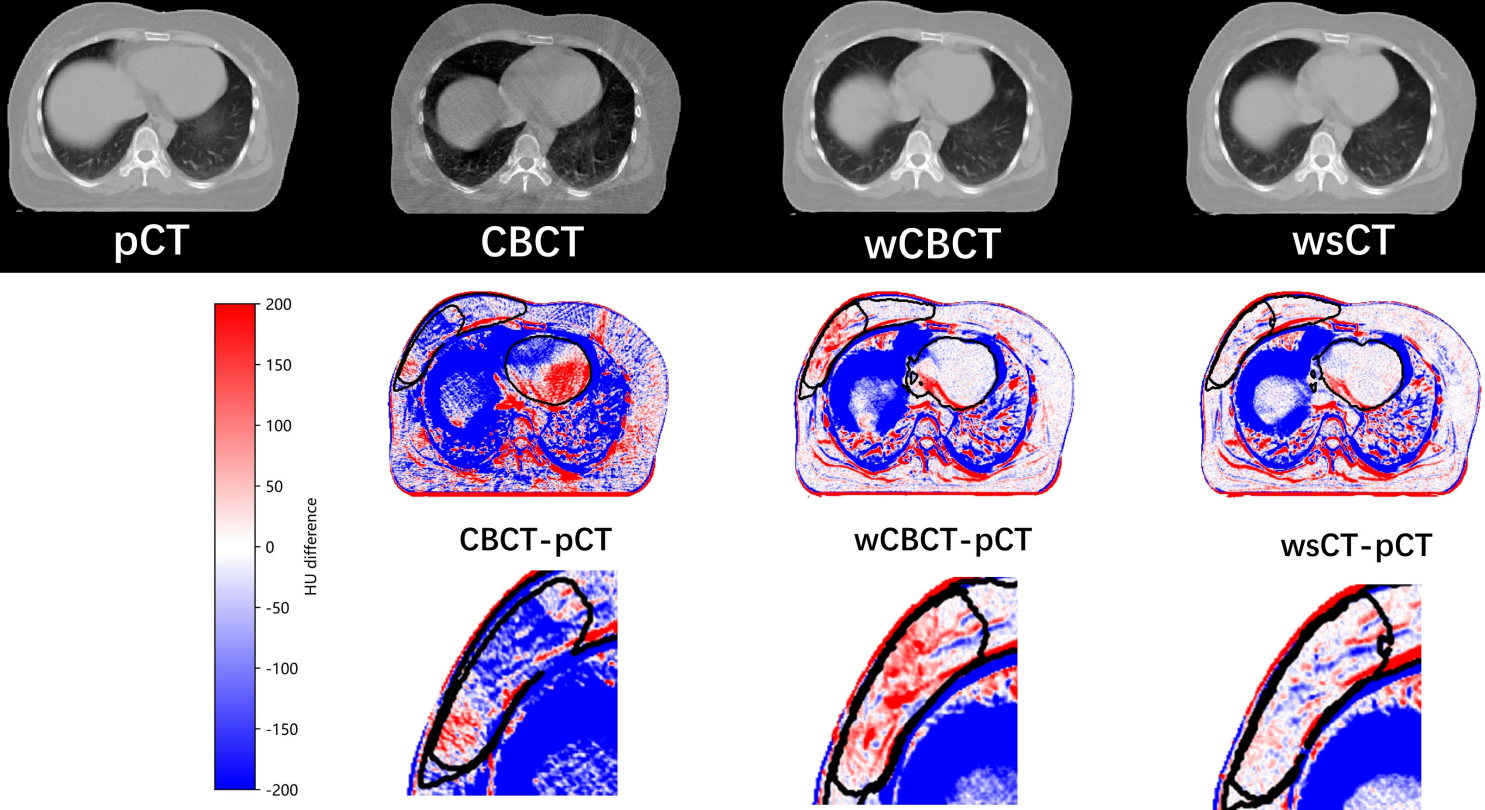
The pCT, CBCT, warped CBCT, and warped sCT images displayed in the same window for a single patient, and the second row shows the difference between CBCT, warped CBCT, and warped sCT images and pCT images.
